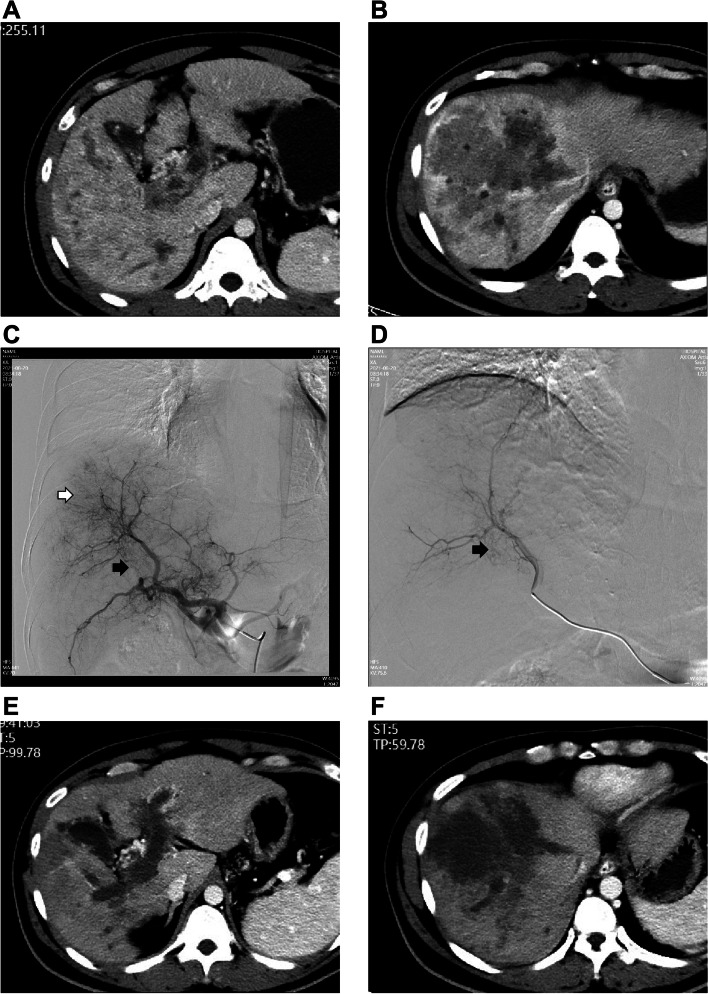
Superselective embolization TACE procedure and follow-up in A 40-year-old male HCC patients with mPVTT. A, B contrast-enhanced CT scan showed a HCC lesion (maximal diameter of 110 mm) with main portal vein invasion. C DSA of common hepatic artery during the first DEB-TACE procedure (Black Arrow: mPVTT, White Arrow: Tumor leision). D superselective embolizations of tumor feeding artery during DEB-TACE procedure (Black Arrow: mPVTT). E, F contrast-enhanced CT scan showed PR in 1 month follow-up. mPVTT = main portal vein tumor thrombus; DSA = Digital subtraction angiography; DEB-TACE = Drug Eluting Beads-TACE
